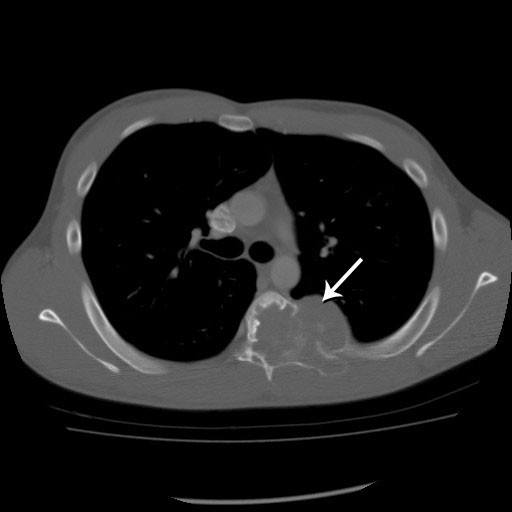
Pre-operative axial computed tomography (CT) windowed for bone at the level of T6 shows an osteolytic and expansile lesion predominantly involving the left vertebral body, posterior elements, and proximal rib with a large intra-thoracic soft tissue component.
